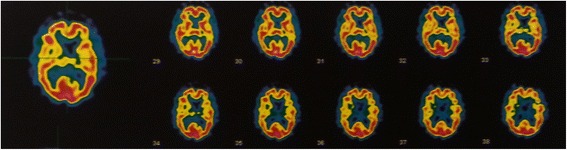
Brain ^99^mTc-hexamethylpropyleneamineoxime single-photon emission computed tomography (SPECT) imaging shows moderate hypoperfusion in both occipital lobes, and associated with marked decreased perfusion in parieto-fronto-temporal lobes bilaterally. SPECT images were generated in the transaxial plane, with direction of the slices from feet to head
